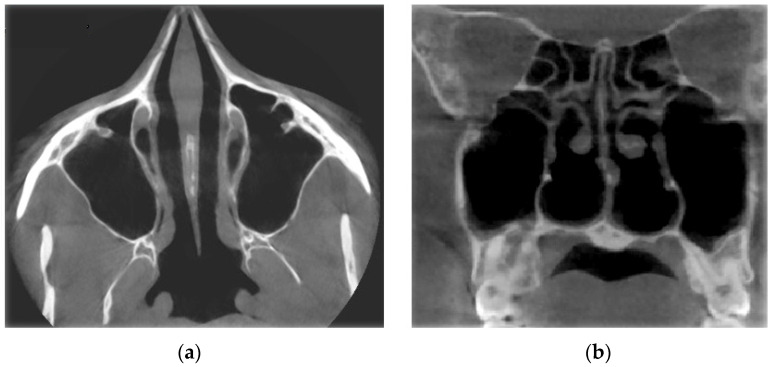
Computed tomography of a patient with empty nose syndrome after conchotomy—bilateral removal of the lower shells: (a) axial tomographic section; (b) multiplanar reconstruction in frontal projection.
